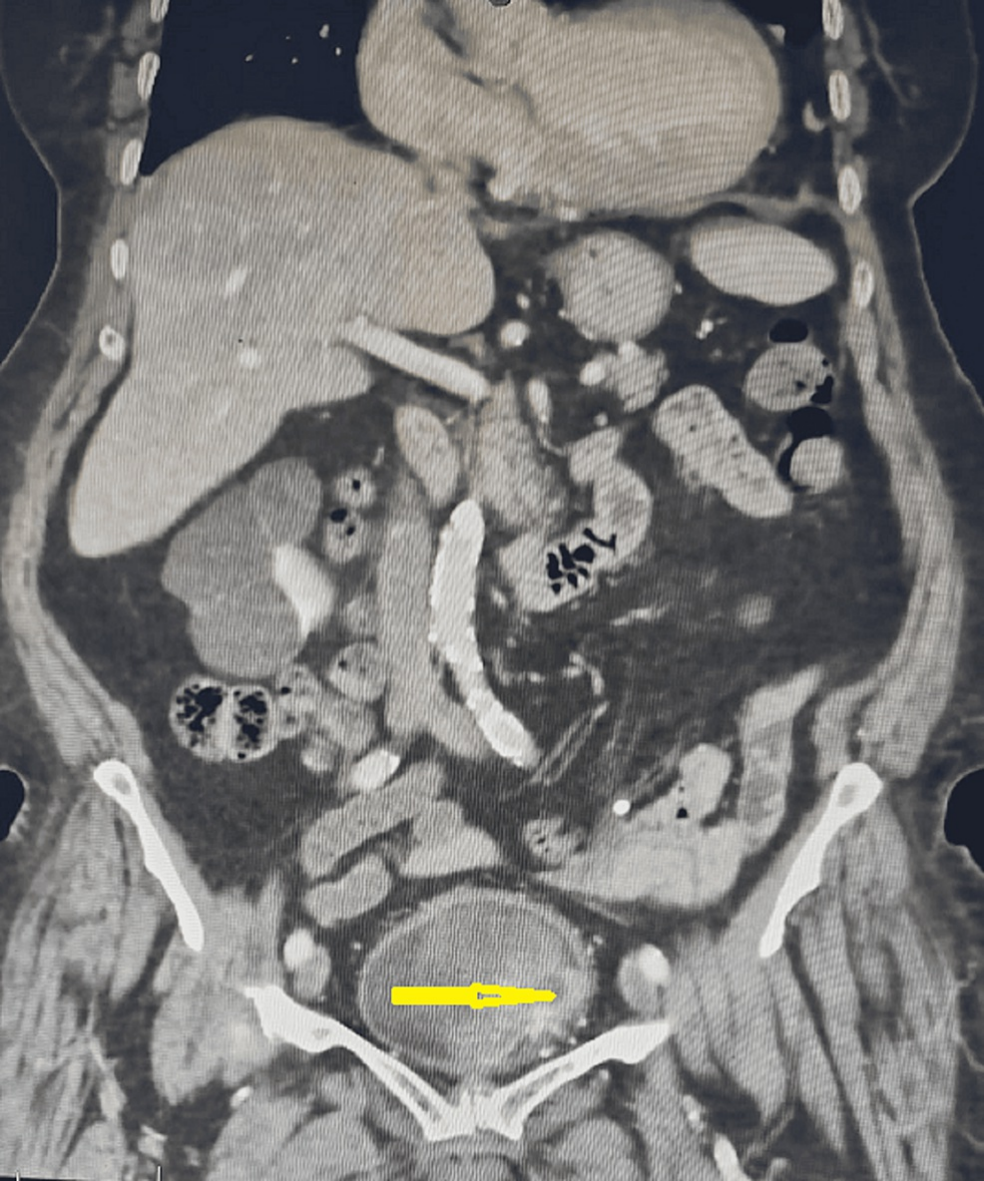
An abdominal CT scan with contrast revealed a 6.2 × 6 cm heterogeneous mass in the left wall and base of the bladder (arrow) CT: computed tomography
